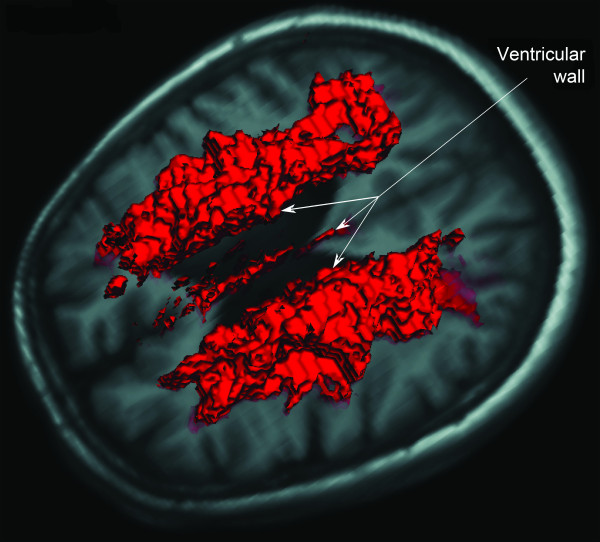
Visualization of chronic white matter lesions obtained by MRI. The hyper-intense areas in the FLAIR images (in red) are overlain onto the T1 images and shown in three dimensions. The ventricular walls (arrows), adjacent periventricular and subcortical hyper-intensities are visualized.
